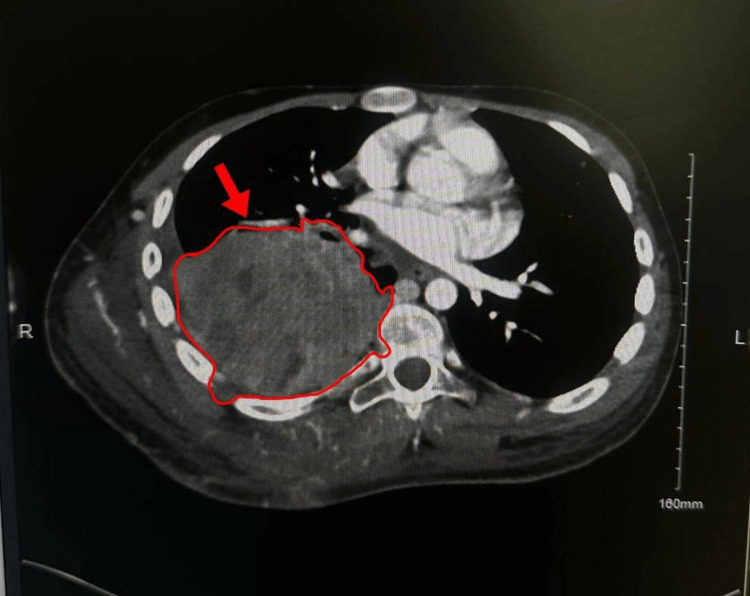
An axial contrast-enhanced CT of the thorax showing the large heterogenous right posterior-inferior chest-wall mass compressing the right atrium and extending into the spinal canal at T12/L1
